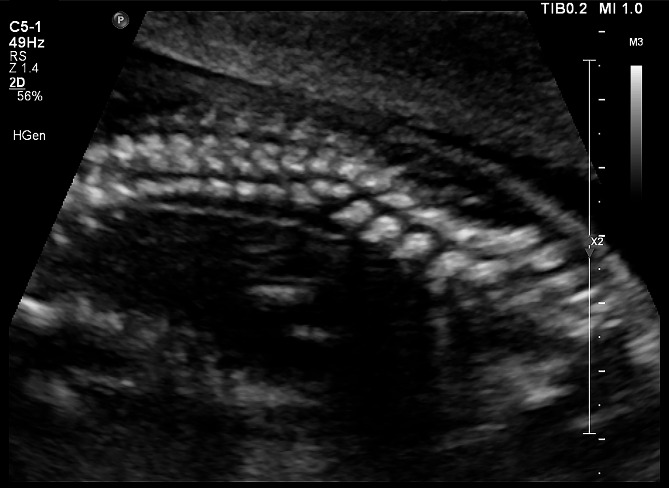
Ultrasound scan of the fetus at 17 weeks of gestation
